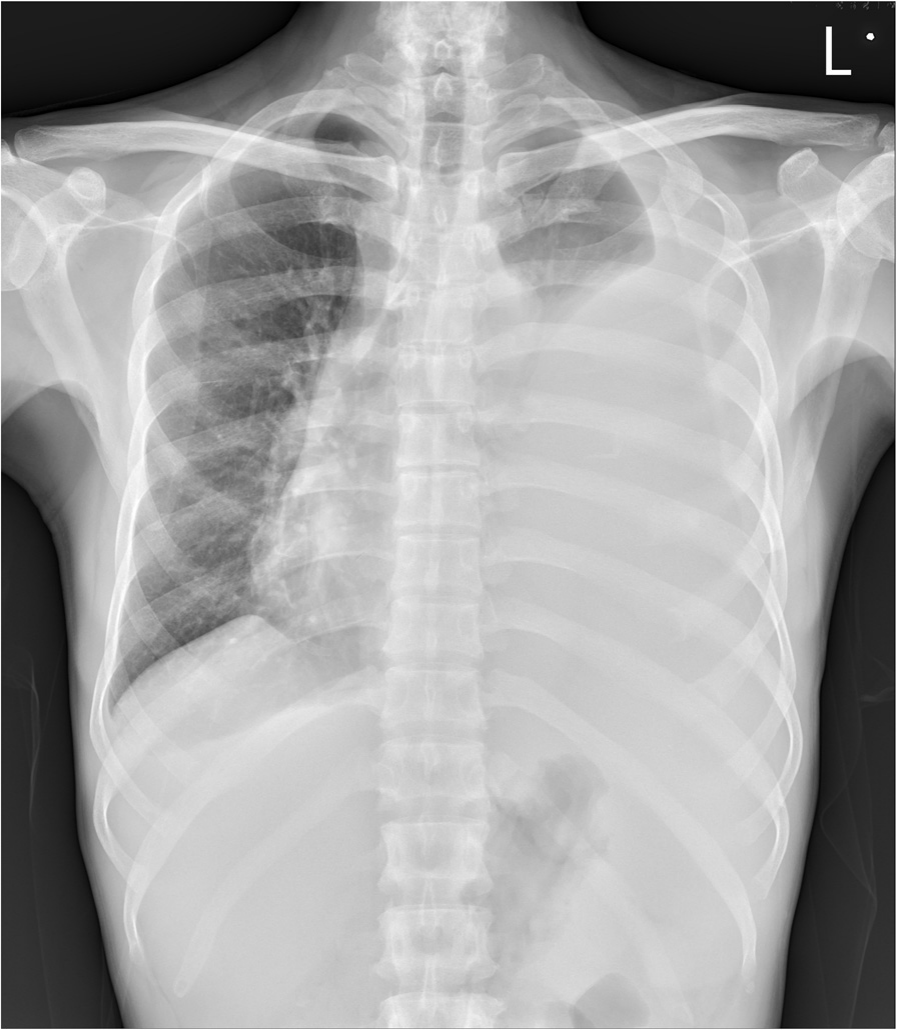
Chest radiograph reveals massive left-sided pleural effusion with a right-deviated trachea
